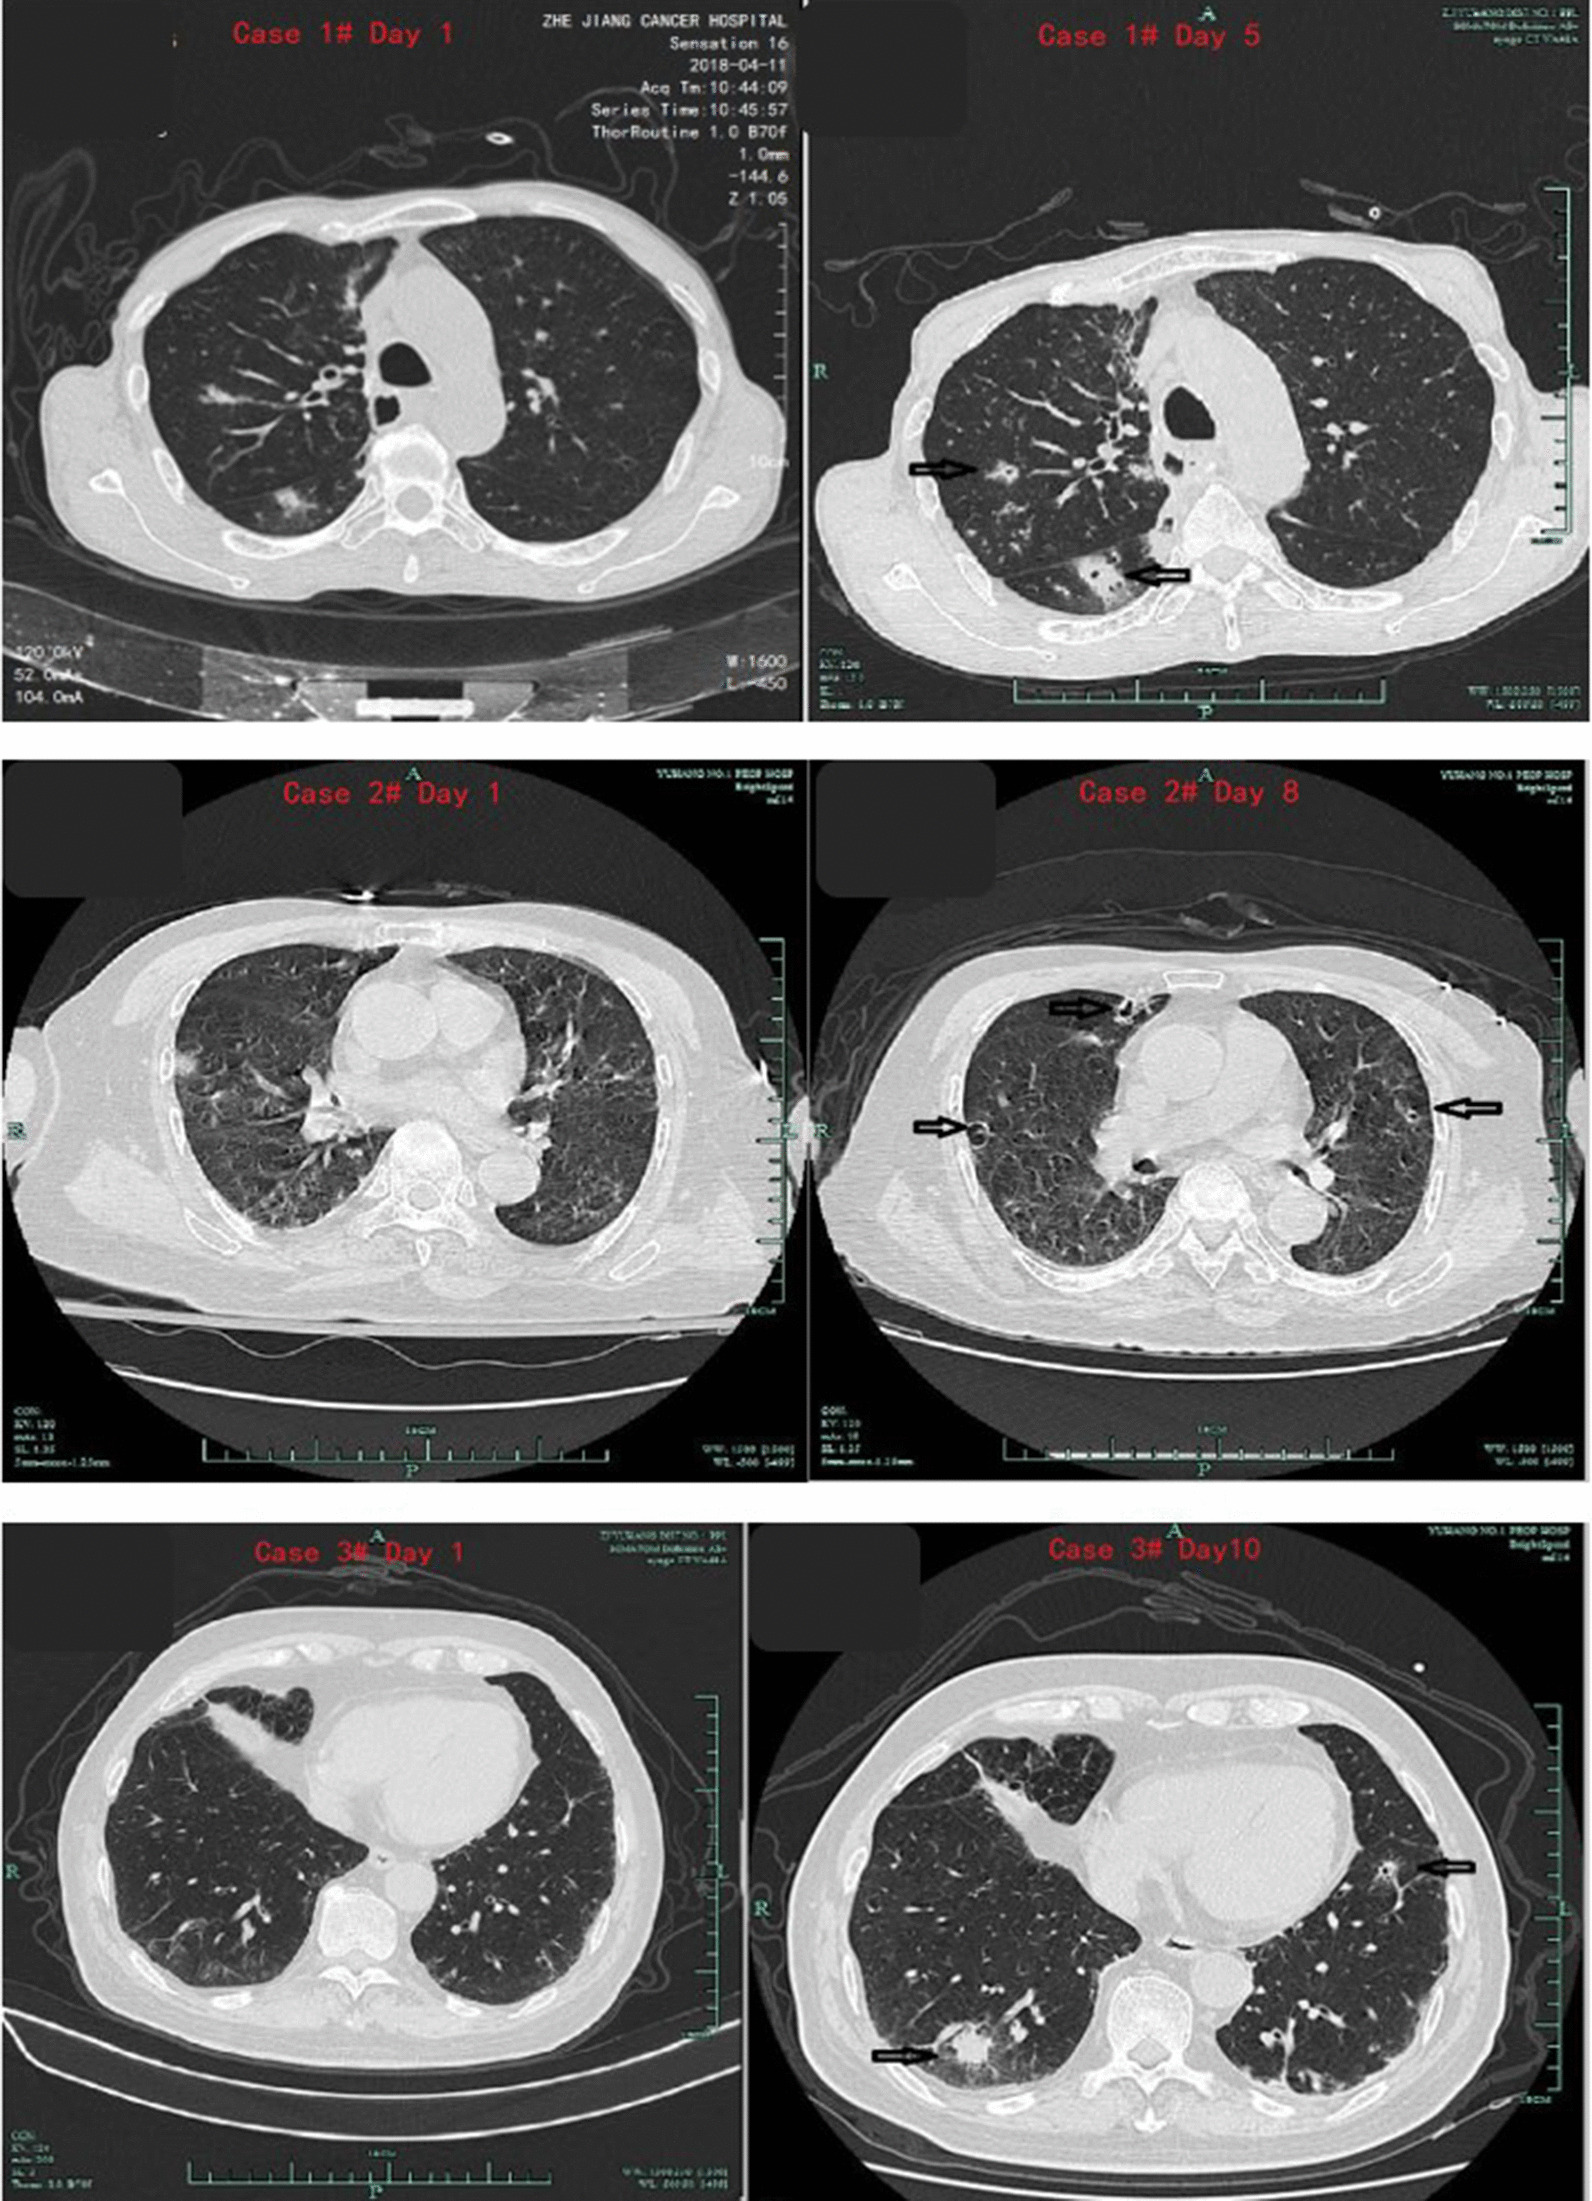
Dynamic monitoring of CT scans at short intervals of 5, 8 and 10 days in three cases respectively. The first CT scan of patients showed nonspecific signs. The second CT scan had deterioration with multiple nodules and cavities. The black arrows showed the specific signs of nodular and cavity
